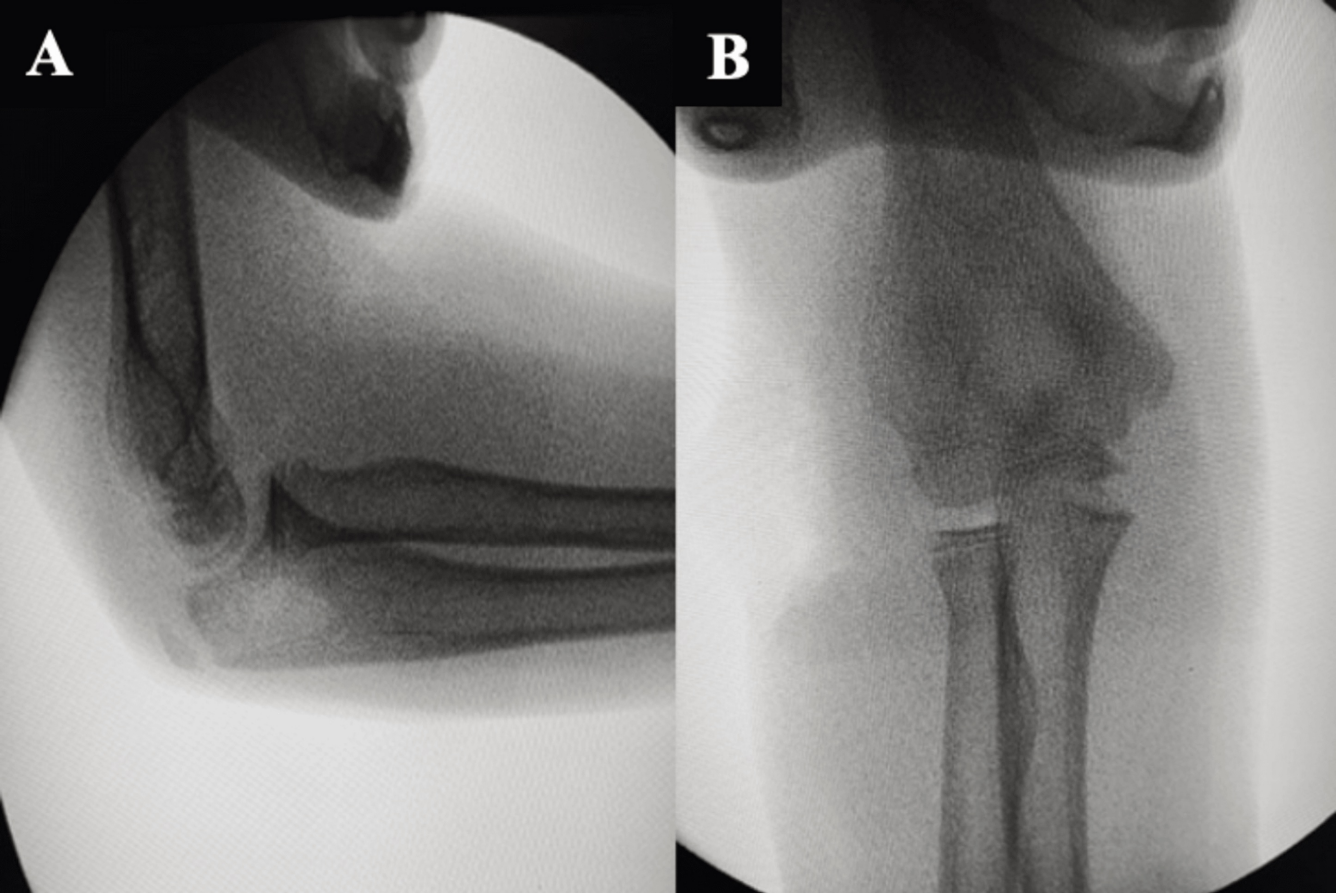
X-ray showing the intra-operative reduction of radial head dislocation of the right elbow. 3A shows the lateral radiographic view of the right elbow; 3B shows the anteroposterior radiographic view of the right elbow.
